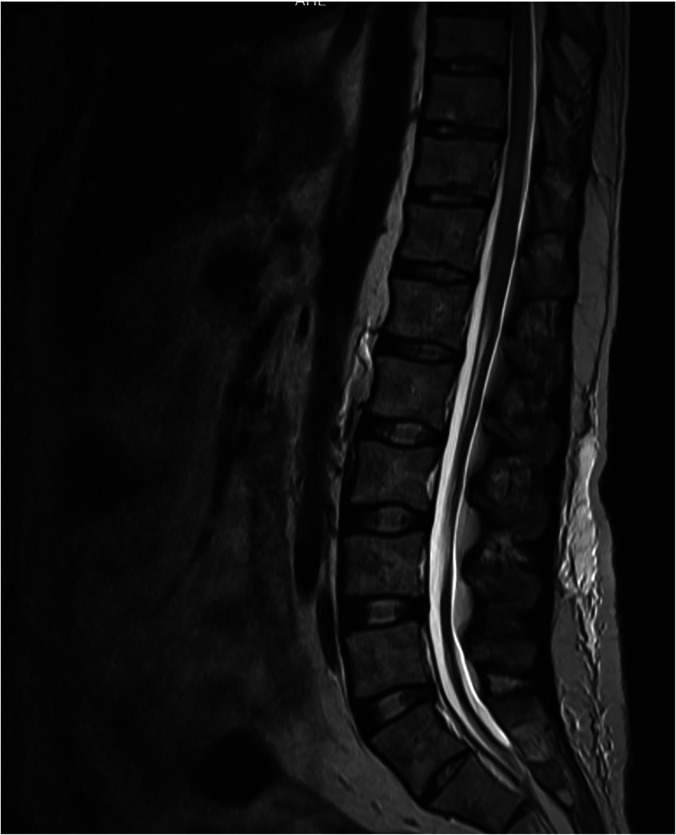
Progress MRI with contrast after 3 months of treatment of neuroschistosomiasis with praziquantel and pulsed methylprednisolone, followed by a tapering course of prednisolone. Compared with the initial MRI, the hyperintense T2 signal has decreased at T8–T12 and no longer affects the conus medullaris, and the edema of the conus medullaris has resolved.
